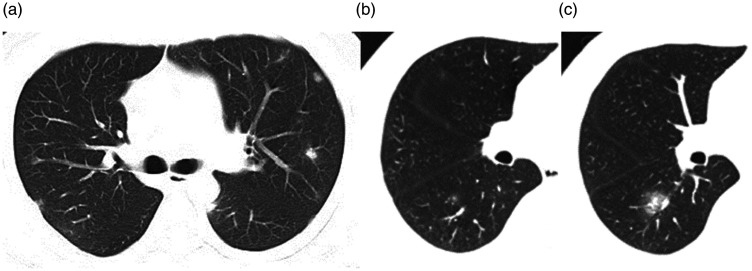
(a) Case 5 14 days after onset of symptoms. GGO mimics nodules in the bilateral lung. (b, c) Case 2. (b) CT scan one the day of onset of symptoms, (c) CT scan four days after onset of symptoms. A GGO mimicking a nodule is seen in the dorsal segment of the right lower lung in (b). The lesion progresses in (c).
